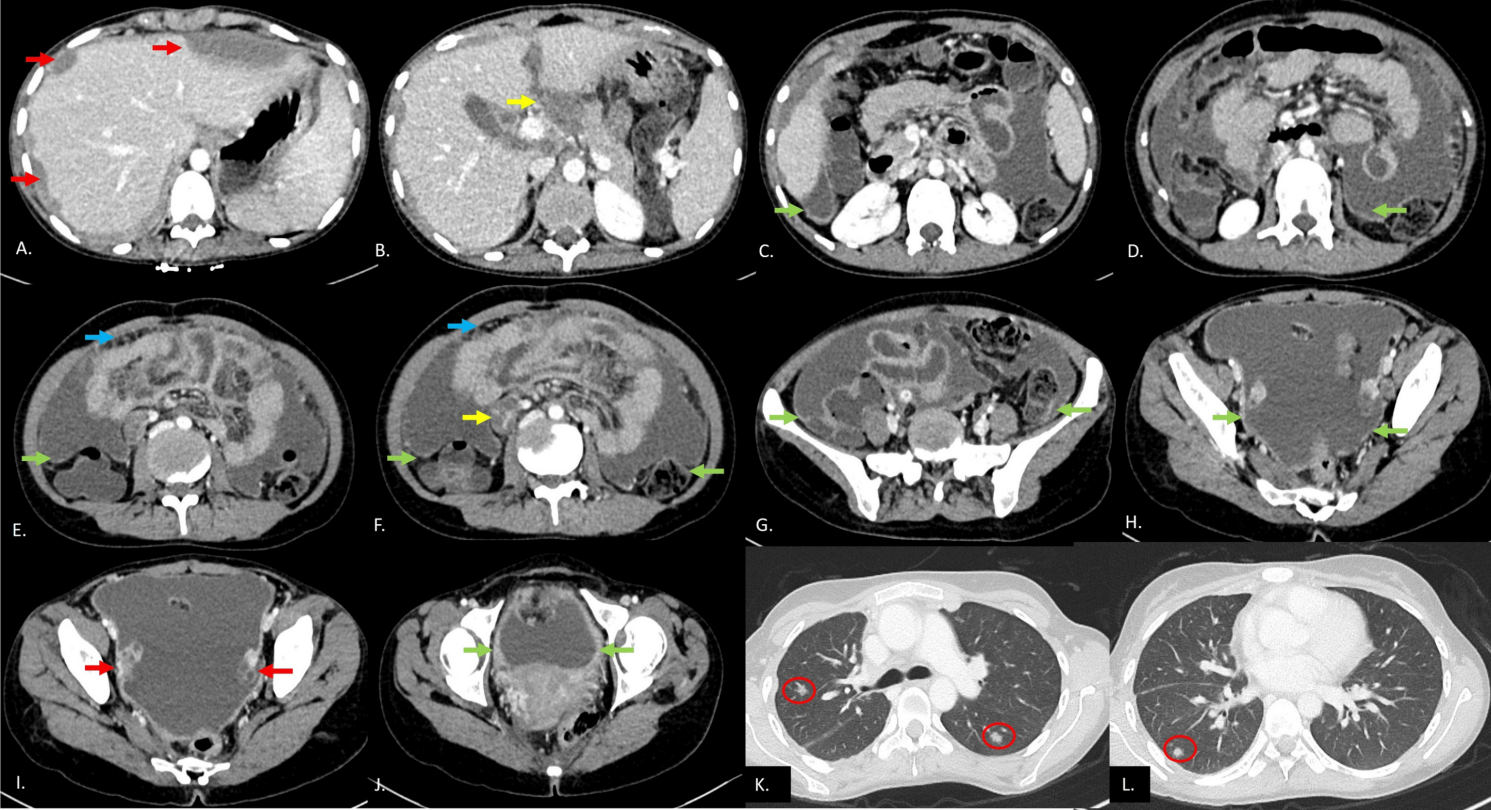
Sequential axial CECT abdomen images shows (A) scalloping of liver margins due to subcapsular collections in liver (red arrows), (B) heterogeneously enhancing hypodense lesion in region of porta infiltrating the body of pancreas (yellow arrow), (C)-(J) smooth peritoneal thickening (green arrows in (C)-(J)) with mild omental thickening (blue arrow in (E)) and necrotic mesenteric nodes (yellow arrow in (F)) and bilateral bulky ovaries (right > left) (red arrows in (I)). (K) and (L) Axial lung window sections show focal nodular consolidations in bilateral lung (red circles). CECT: contrast-enhanced computed tomography.
